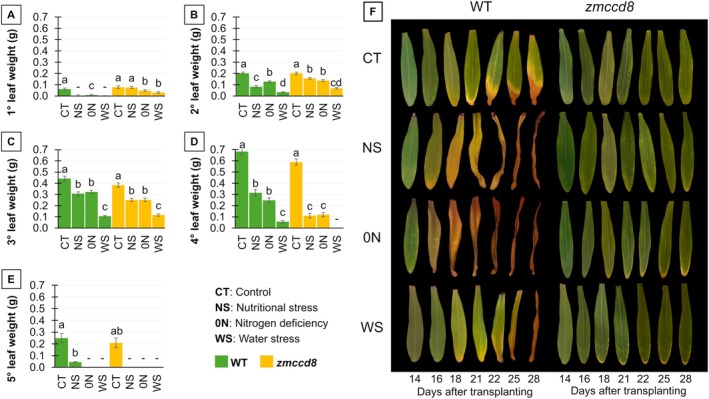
Fresh weight of the first (A), second (B), third (C), fourth (D), and fifth (E) leaves of WT (green) and *zmccd8* (yellow) maize plants after 4 weeks of growth in vermiculite under different conditions: Control (CT), complete nutrient deprivation (NS), nitrogen deficiency (0N), and water stress (WS). Error bars represent mean ± SE (*n* = 15). Statistical analysis was performed using one‐way ANOVA followed by Tukey's HSD test. Different letters indicate statistically significant differences (*p* < 0.05). (F) First leaf phenotype of WT (left) and *zmccd8* (right) seedlings during the final 2 weeks of growth.
